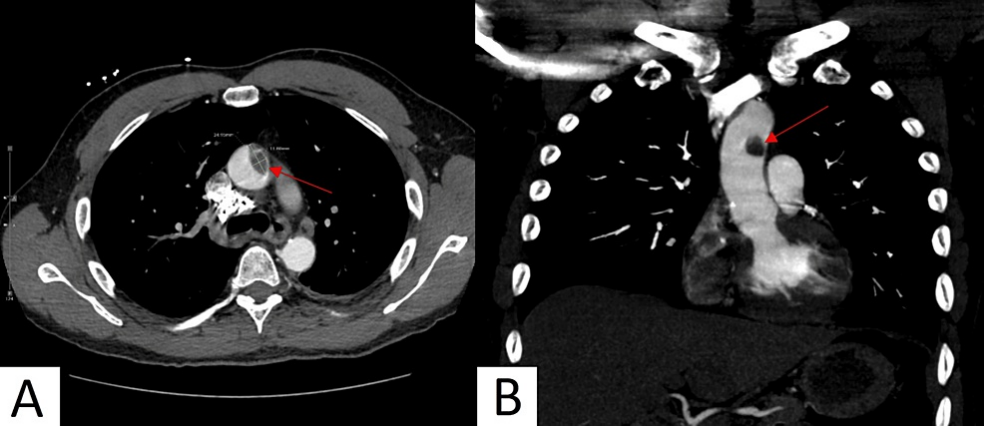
Axial (A) and coronal (B) section of CT pulmonary angiogram. Prominent irregular mural thrombus measuring 2.5 x 1.2 cm in the ascending aortic arch (red arrow).
